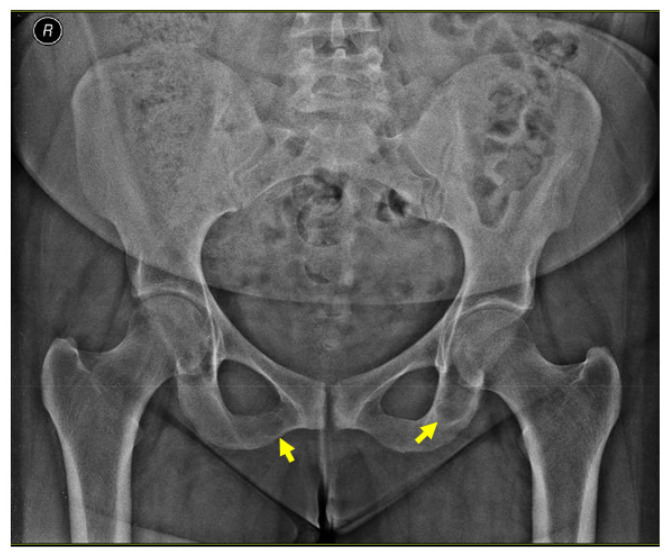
X-ray of the pelvis: brown tumors at the level of the ischium (yellow arrows).
